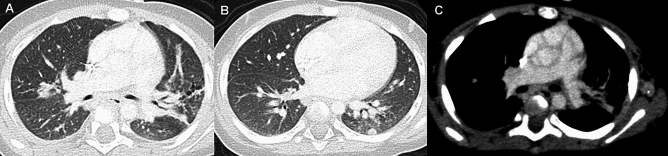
Chronic granulomatous disease. An 11-month-old boy. A,B CT showed various inflammatory changes, including infiltrative shadows, nodules, masses, and GGOs along with local emphysematous changes in both lungs. C Mediastinal, hilar, and axillary lymphadenopathy was evident as well
